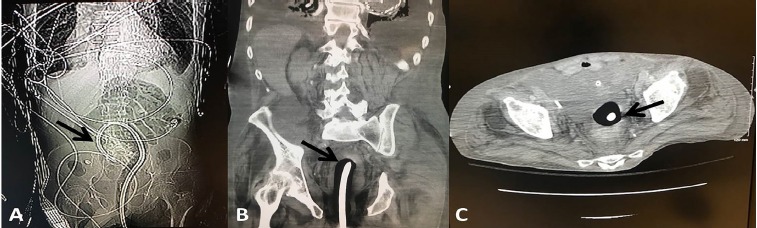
Radiologic images of balloon tamponade placement with arrows indicating the inflated Minnesota tube: A) radiograph showing the full placement; B) computed tomography (CT) of coronal plane showing placement in the descending colon; C) CT of axial plane showing placement in the colon.
